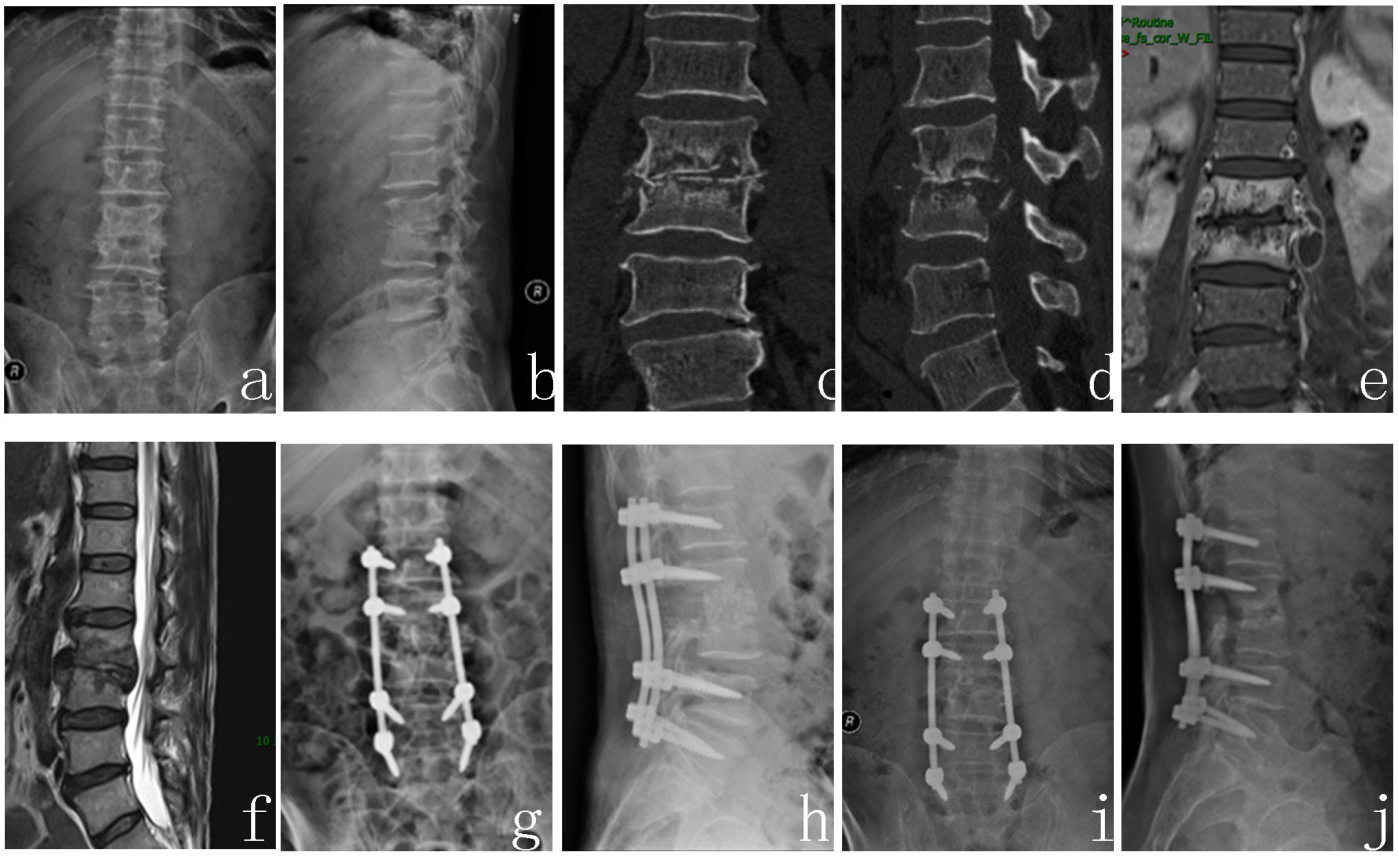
A 58-year-old male patient with L2-3 spinal tuberculosis (Frankel grade D) achieved autogenous iliac bone graft for reconstruction. (a~f) Preoperative X-ray, CT, and MRI showed that L2 and L3 vertebra body and the intervertebral disc were destroyed to produce neurological compression and instability. (g, h) Postoperative X-ray. (I, j) A-P and lateral X-ray at 29 months after surgery showed good location of titanium mesh, lumbar physiological curve and neurologic function recovery.
